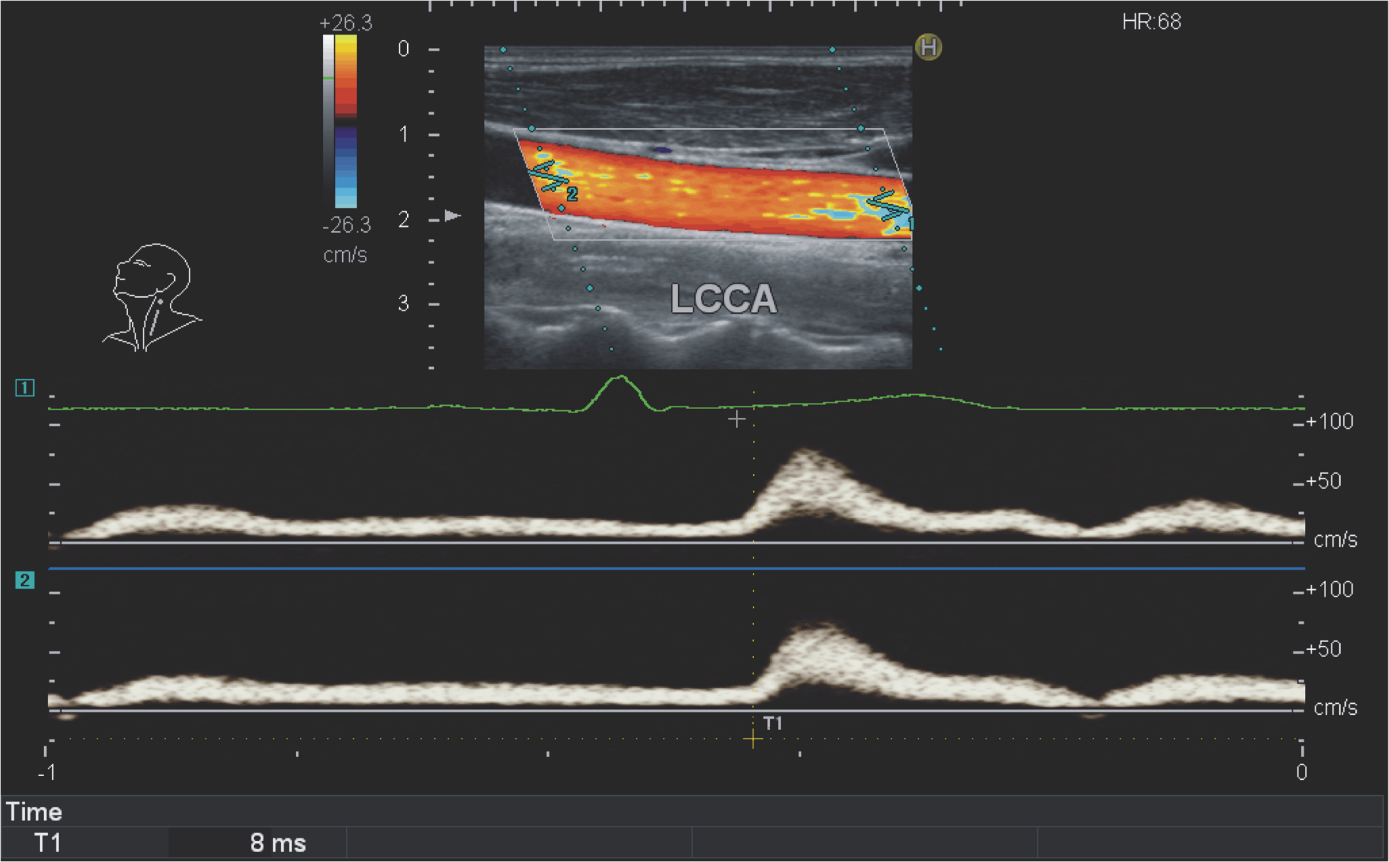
A representative local PWV measurement in a healthy subject. In the upper part, the two dimensional echography showed the upstream sample volume 1 and downstream sample volume 2 and their exact distance could be measured. The Doppler simultaneous velocity spectra were shown at the lower part. The transit time between these two sites was measured (8ms). LCCA, left common carotid artery; PWV, pulse wave velocity.
